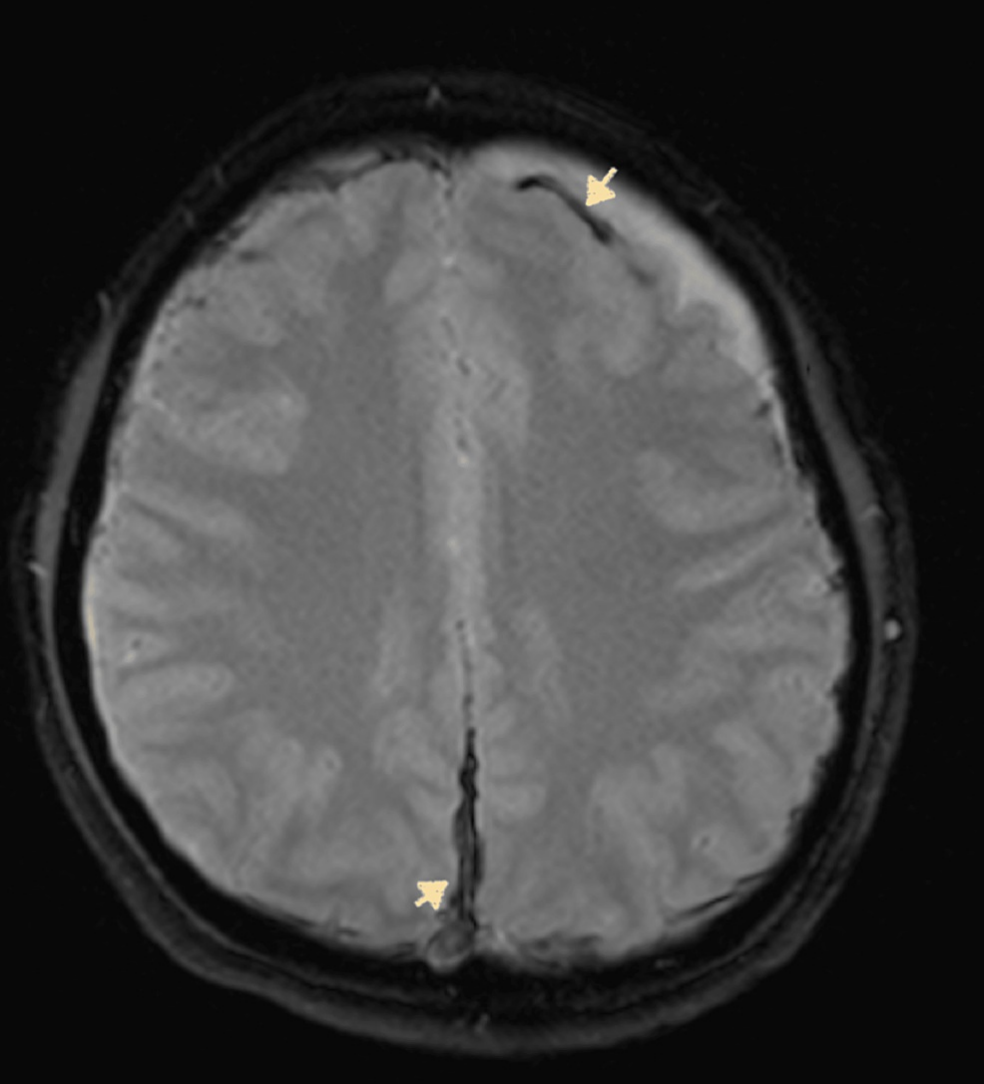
MRI GRE sequence shows subdural hematomas in the frontal cortex and midline falx (white arrows). MRI: magnetic resonance imaging, GRE: gradient recalled echo
